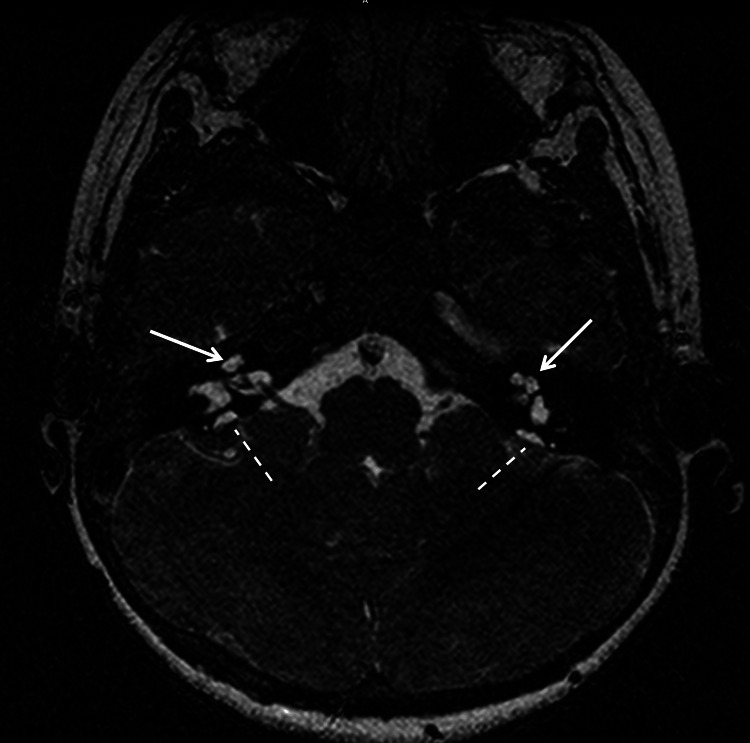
MRI of the brain showing bilateral malformed cochleae (solid arrows) and endolymphatic sacs (dotted lines), both representing Mondini’s malformation
